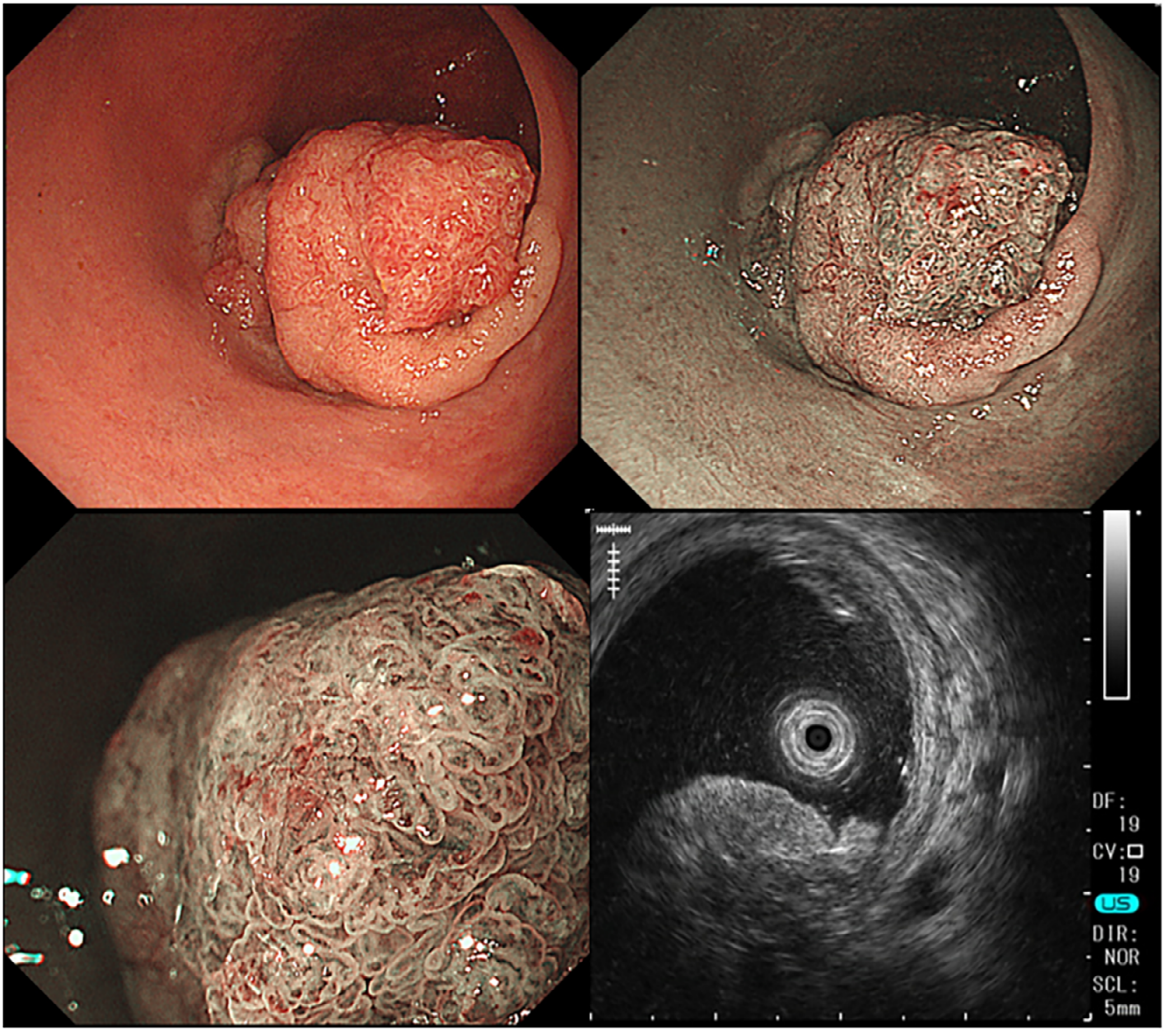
Endoscopic and endoscopic ultrasound assessment of an elevated colorectal cancer lesion. Although surface evaluation alone was insufficient to confirm deep invasion due to the absence of exposed highly atypical areas, endoscopic ultrasound assessment confirmed muscularis propria invasion, leading to a decision for surgical treatment. Pathological examination confirmed a pT2 depth of invasion.
